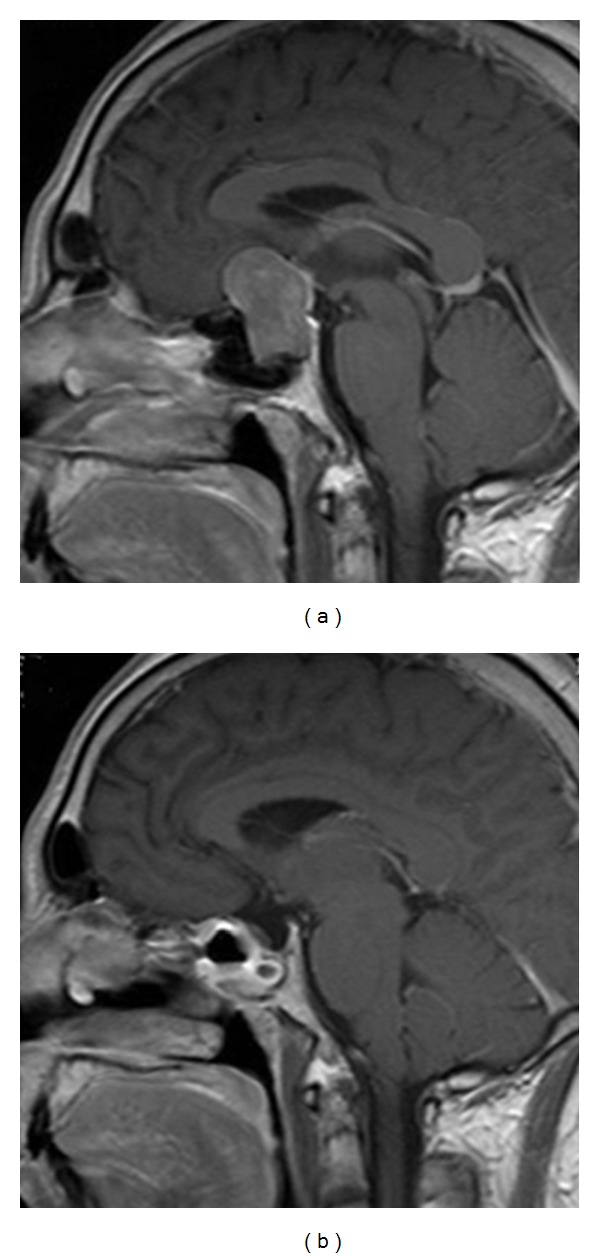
Enhanced MRI sagittal view. In this case, the tumor presented a small portion growing toward the anterior cranial floor (a). An extended TS approach was used and the tumor could be successfully taken out (b).
